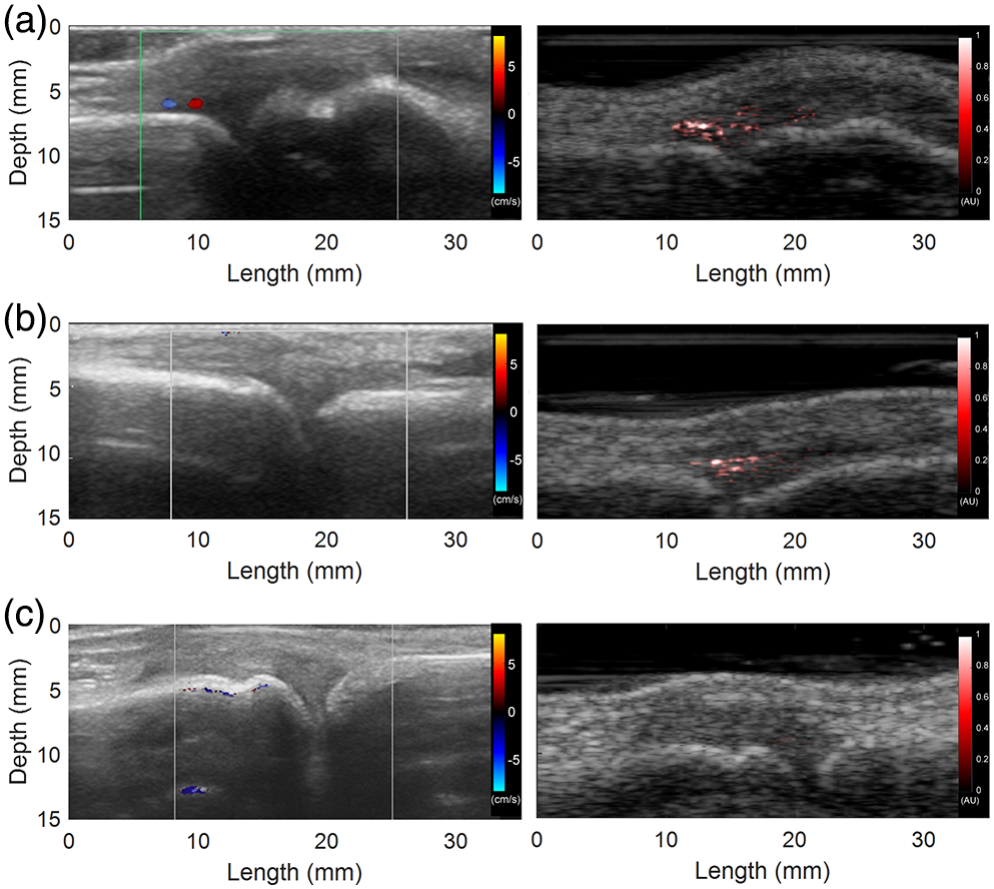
(Left) US Doppler images and (right) PA images of human MCP joints. (a) The images of a clinically active inflammatory arthritis joint showing hyperemia in both US Doppler and PA images. (b) The images of a subclinically active inflammatory arthritis joint showing hyperemia in PA image only but not in US Doppler image. (c) The images of a normal joint showing no hyperemia in either US Doppler image or PA image.
